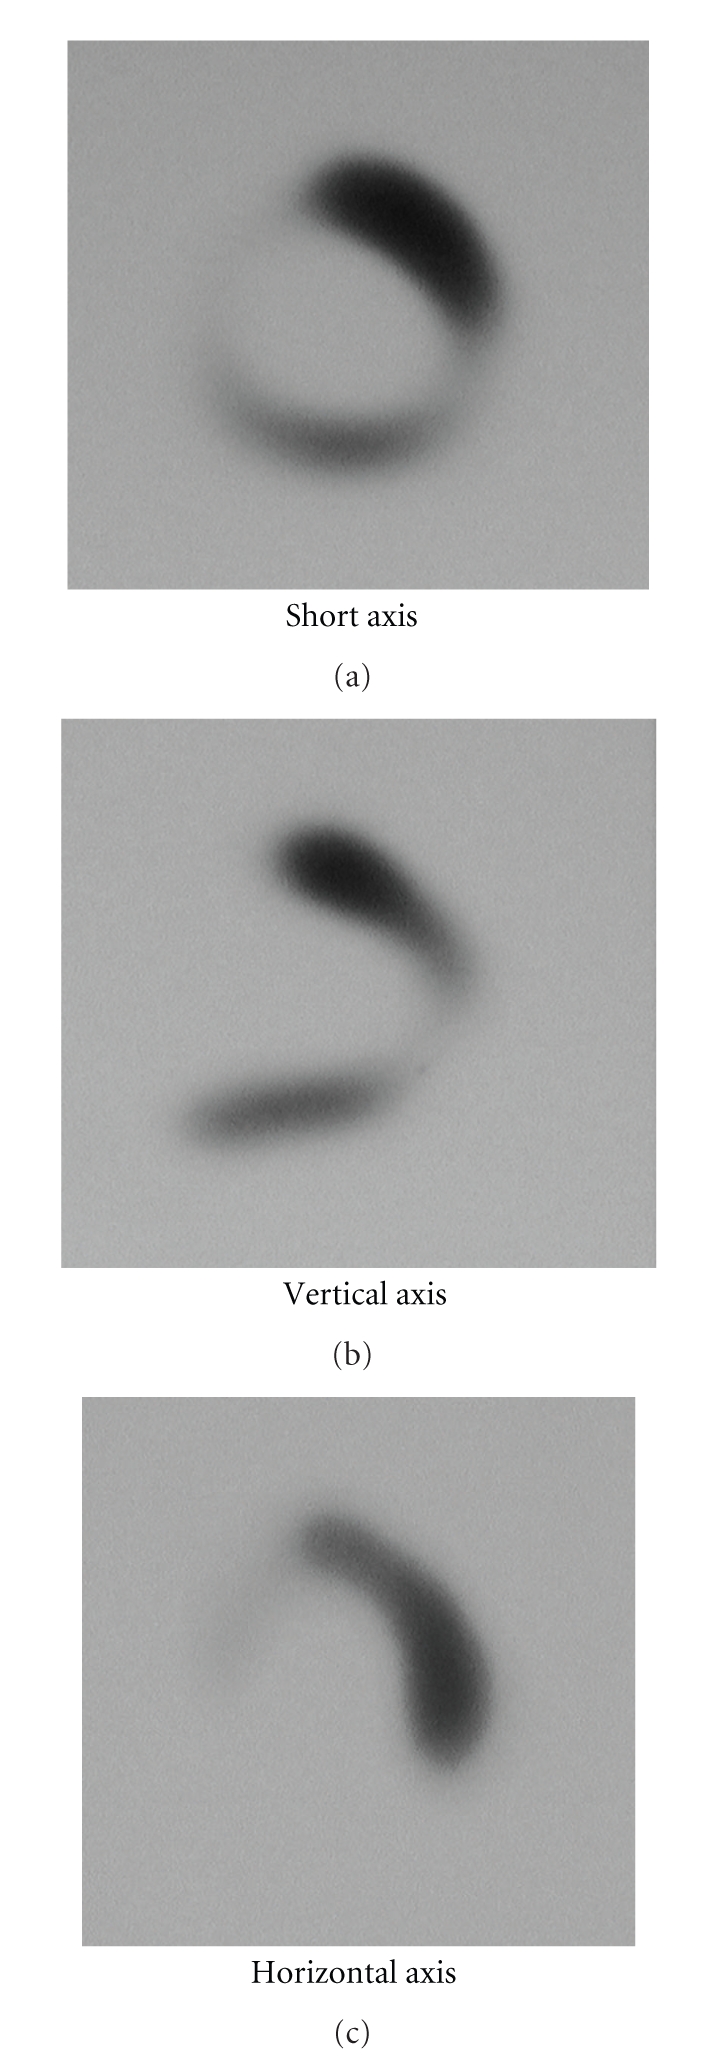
Myocardial scintigraphy with ^99m^Tc-tetrofosmin shows perfusion defect at the septal wall and hypoperfusion at the posterior wall and apex.
